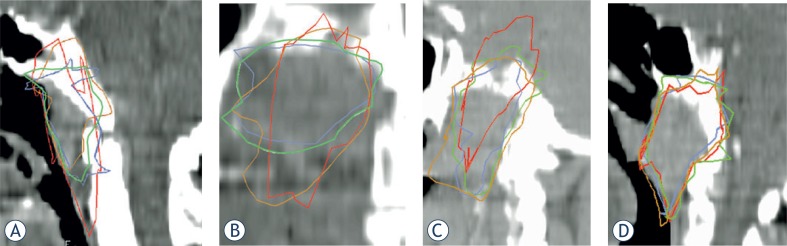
Gross tumor volume (GTVs) based on different MRI sequences fused into sagittal (A, C, D) or coronal (B) CT images. Brown = sagittal-based GTV; blue = axial T2-based GTV; red = coronal -based GTV; and green = axial T1-based GTV.
